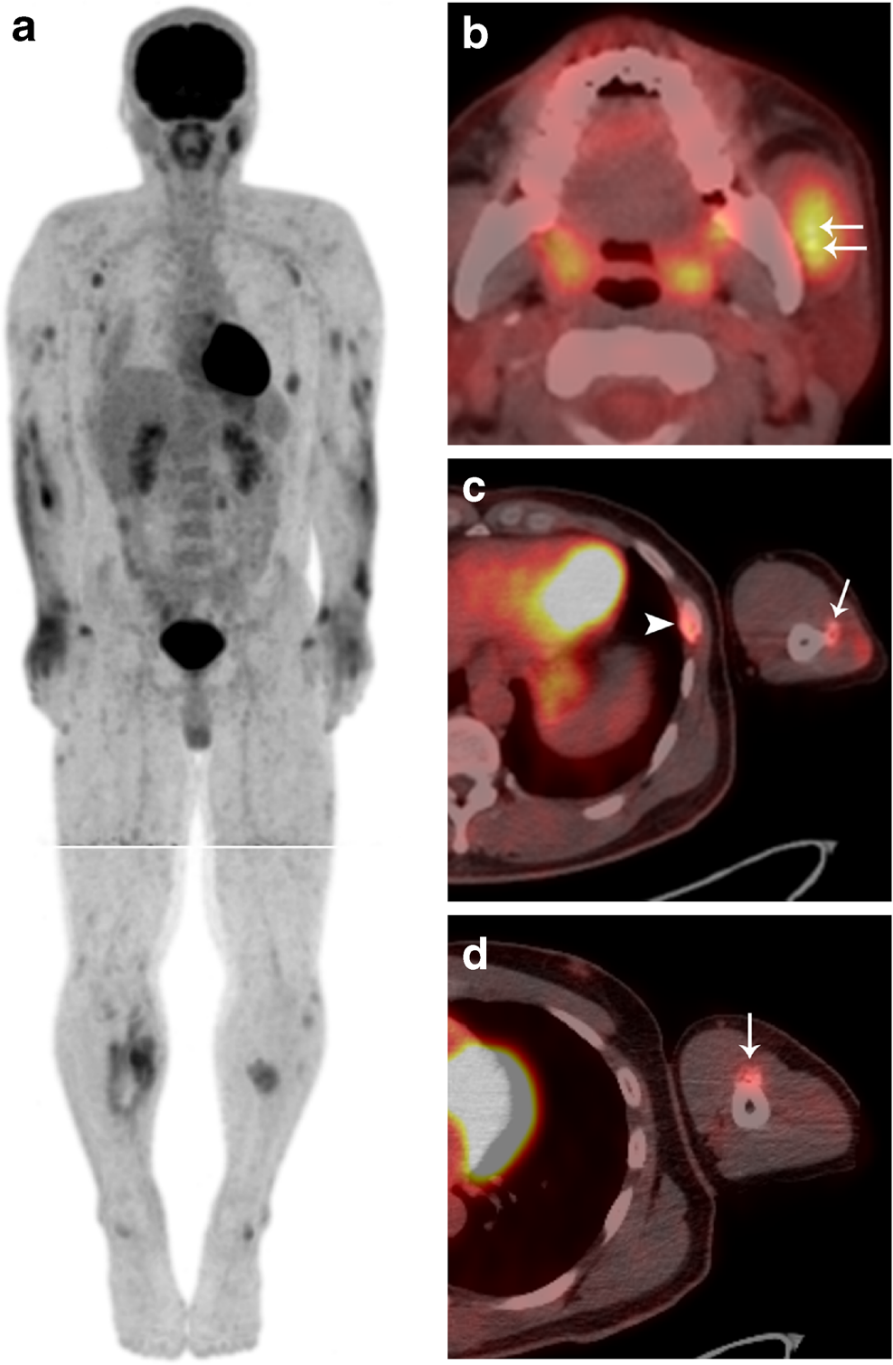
18F-fluorodeoxyglucose (FDG) positron-emission tomography/computed tomography (PET/CT) images. a The maximum intensity projection image shows multiple spotty or diffuse high-uptake regions across the whole body. The maximum standardized uptake values ranged from 2 to 8. In the lower limb, the image was acquired separately at a delayed scan. b, c, d PET/CT fusion images show small calcifications overlapping the FDG uptakes in the left masseter (b, arrows), triceps brachii (c, arrow), and biceps brachii (d, arrow) muscles. The maximum standardized uptake value of the left masseter muscle is 5.6 (b), and those of the left brachial muscles are 2.7 (c) and 2.3 (d), respectively. A fracture is seen in the left 8th rib, overlapping the FDG uptake (c, arrowhead)
